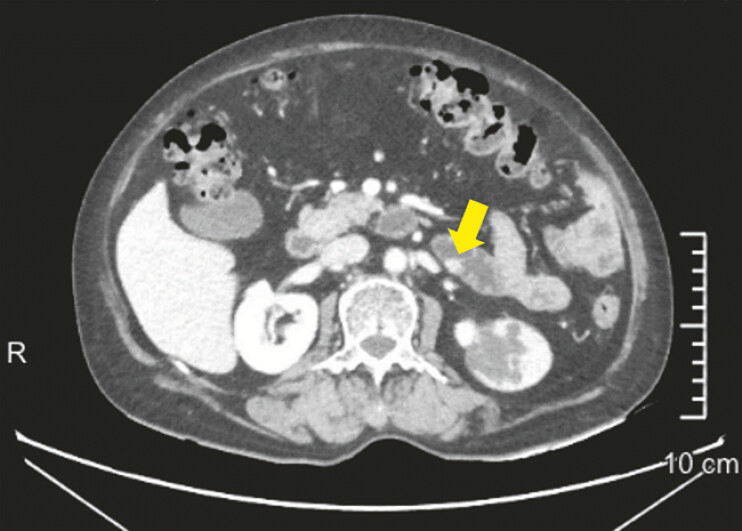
Enhanced CT of the upper abdomen showed a blood-rich nodule on the posterior wall of the proximal jejunum.
